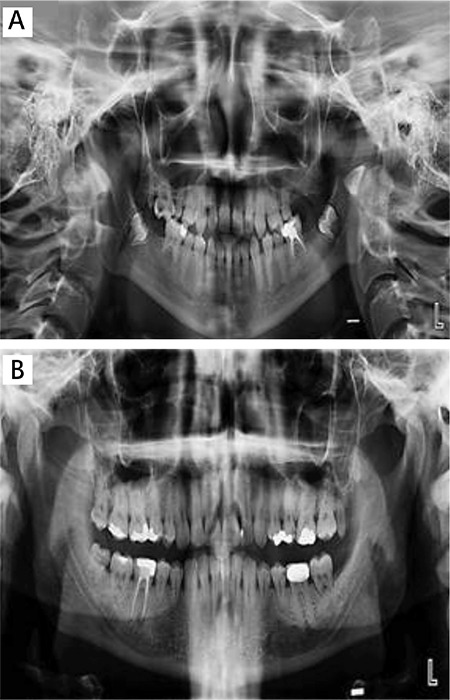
A) Panoramic radiography of case 1; B) panoramic radiography of case 2. A) Panoramic radiography of case 1; B) panoramic radiography of case 2.
